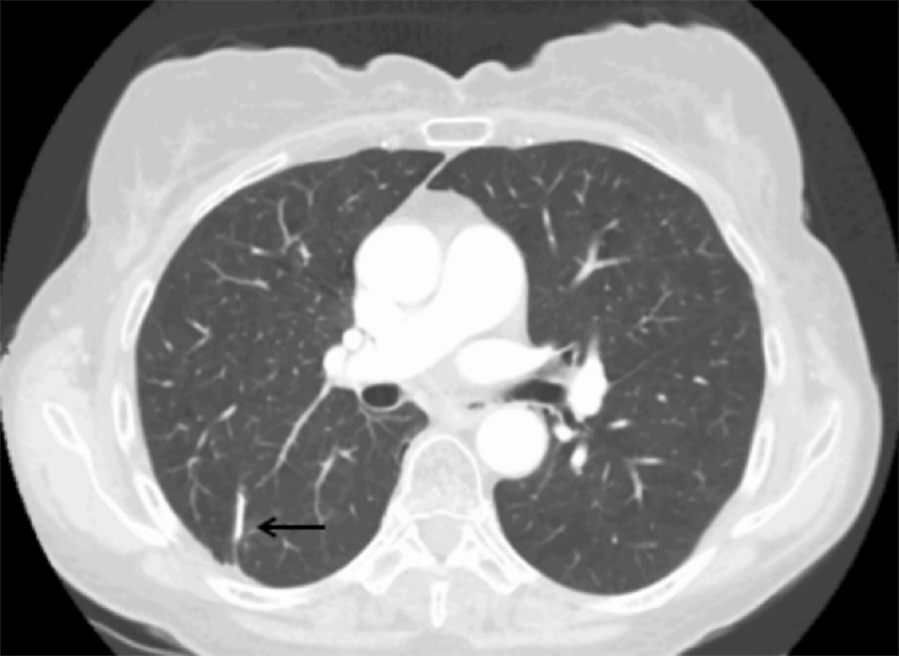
Wedge resection. Axial CT image (parenchymal window) shows a linear increased density due to a parenchymal staple line (black arrow)B.
